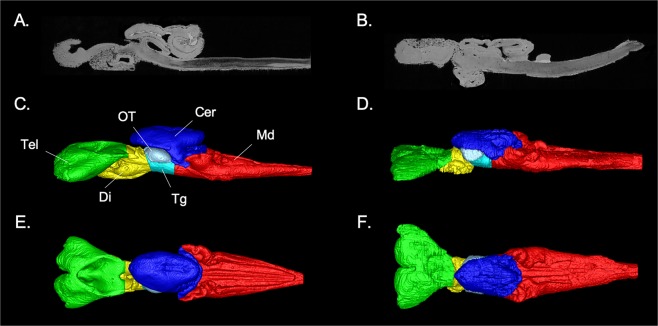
MR images of the brain of the (A,C,E) Greenland shark (*Somniosus microcephalus*) and the (B,D,F) Pacific sleeper shark (*S*. *pacificus*), including a (A,B) sagittal slice of the brain of both species and (E–F) digital segmentation of the major structures of the brain in lateral (C,D) and dorsal (E,F) views. Key: green = telencephalon (Tel), yellow = diencephalon (Di), grey = optic tectum (OT), cyan = tegmentum (Tg), blue = cerebellum (Cer), red = medulla oblongata (Md).
